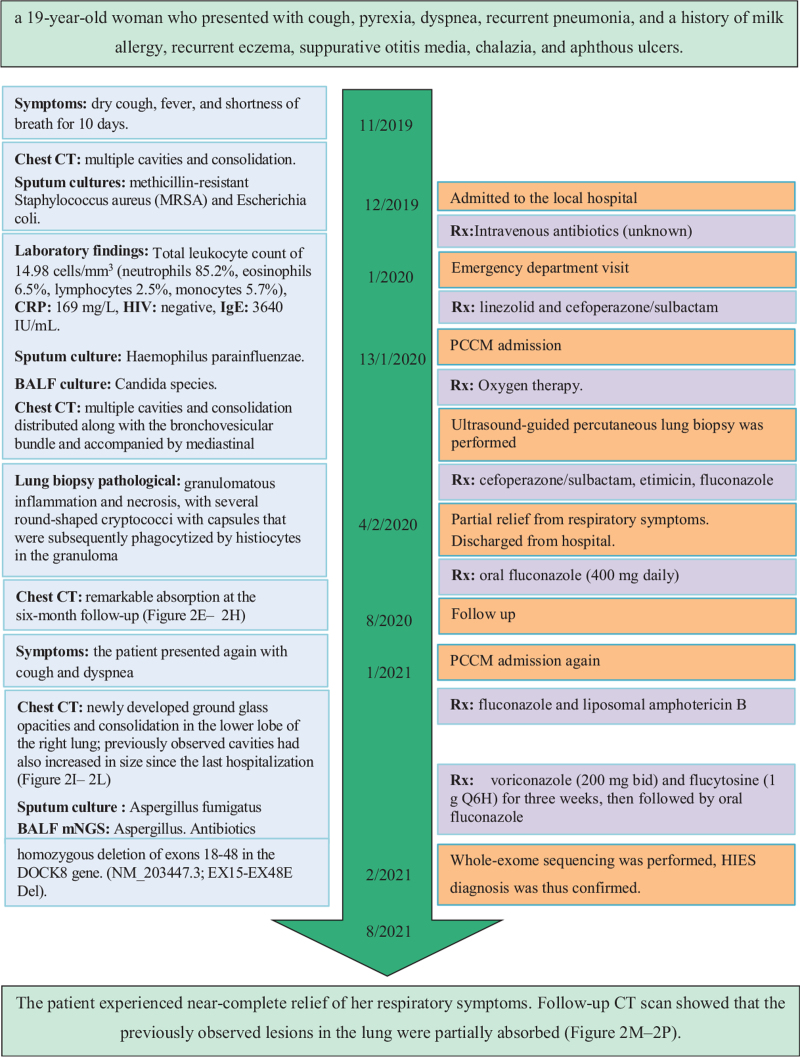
Overview of the patient's clinical presentation and laboratory findings. BALF = bronchoalveolar lavage fluid, CT = computed tomography, CRP = C-reactive protein, DOCK8 = dedicator of cytokinesis 8, HIV = Human Immunodeficiency Virus, IgE = immunoglobulin E, PCCM = pulmonary and critical care medicine.
